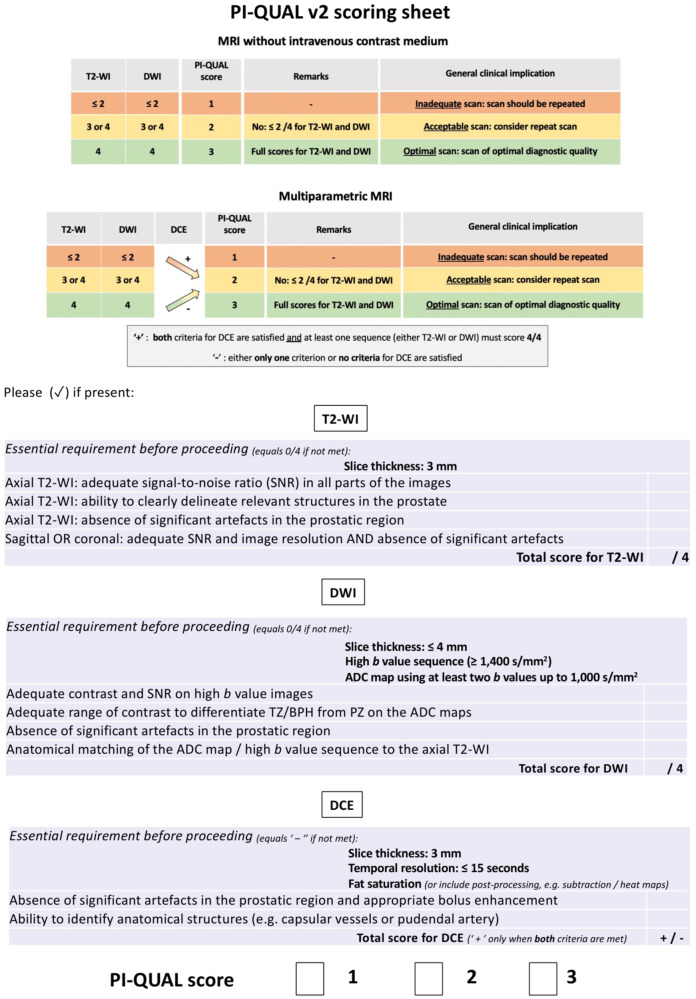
PI-QUAL v2 scoring sheet that includes the basic mandatory PI-RADS v2.1 technical prerequisites for T2-WI, DWI and DCE, and the table to derive the PI-QUAL v2 score
